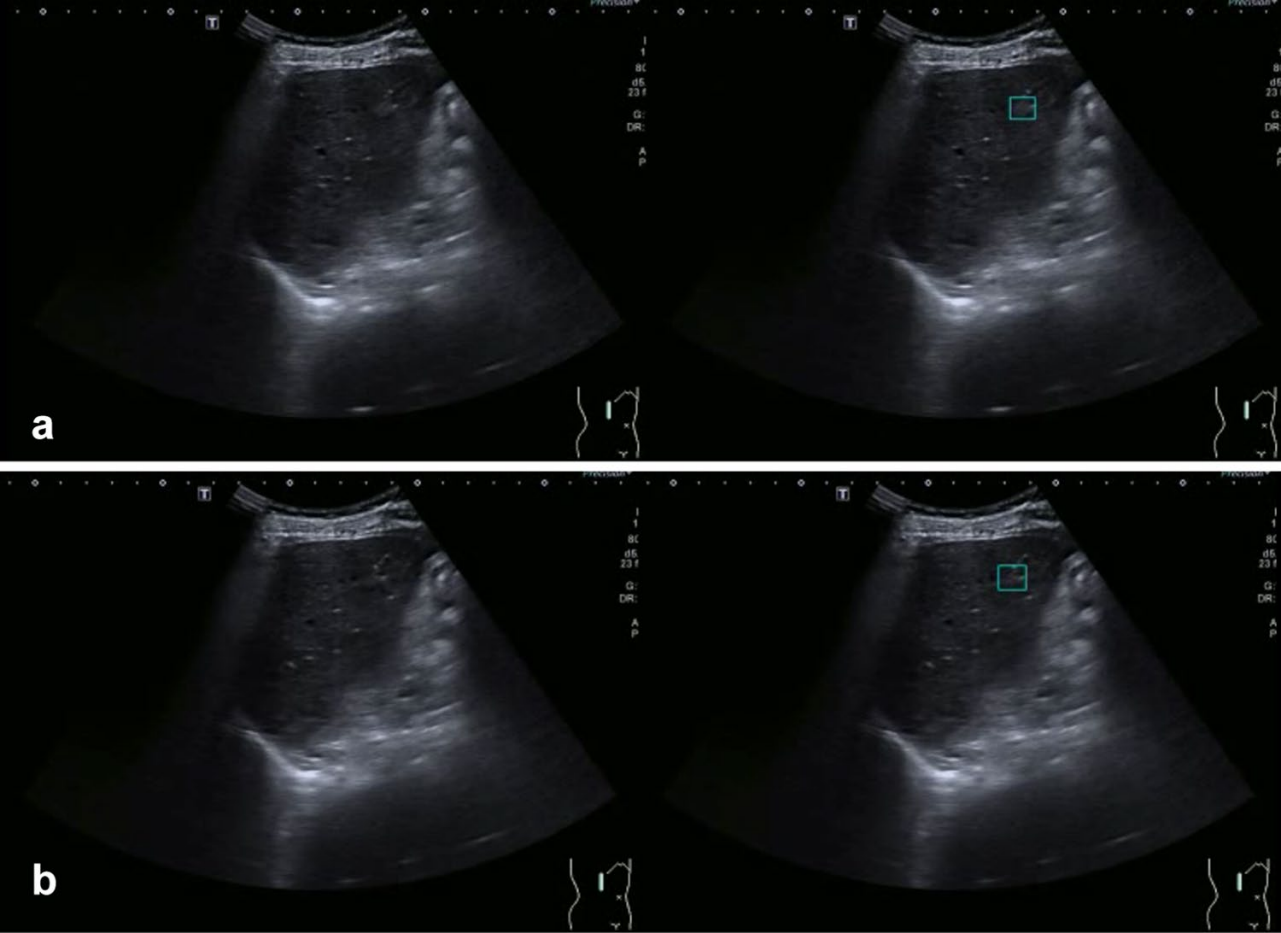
Example of the same lesion appearing as a distinct observation when the ultrasound beam passes through the center of the lesion (2a). In contrast, the lesion appears as a small faint observation when the ultrasound beam passes through the periphery of the lesion (2b). Left panels show the original frames. Right panels show the labeled location of the lesion.
